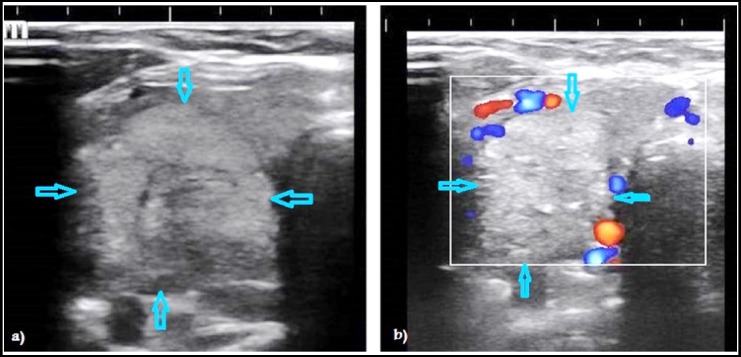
Ultrasound images of thyroid nodules in two different patients. B-mode ultrasonography showing a well-defined round hyperechoic nodule with smooth borders and surrounding halo strongly consistent with benign nodule (a). Power Doppler image (PDI) of another patient showing marginal vascularity around a well-defined round hyperechoic nodule with smooth margins that consistent with benign thyroid nodule but echogenic foci make it indeterminate (b).
